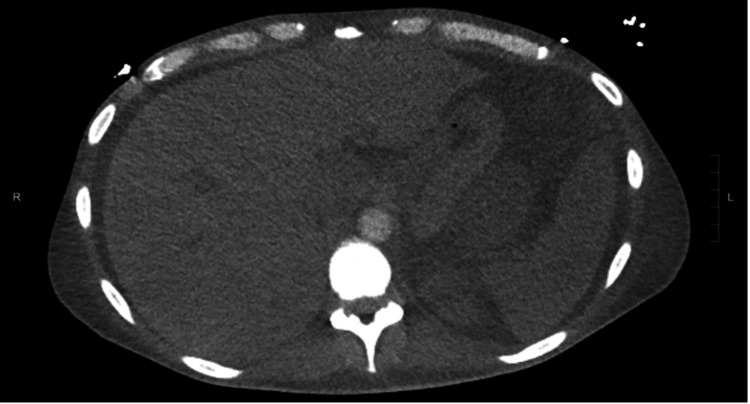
CT scan one month following splenic artery embolization showing no opacification of pseudoaneurysms within the splenic parenchyma
